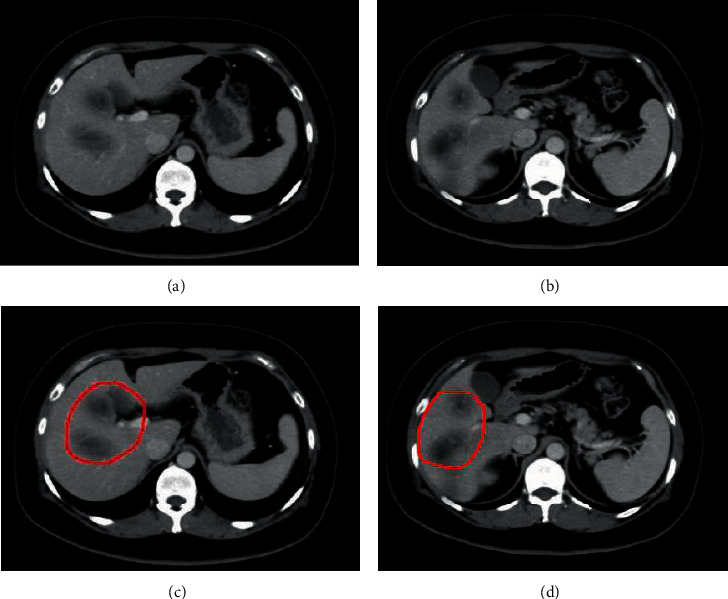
Preoperative CT images of a PHC patient and ROI sketch.
